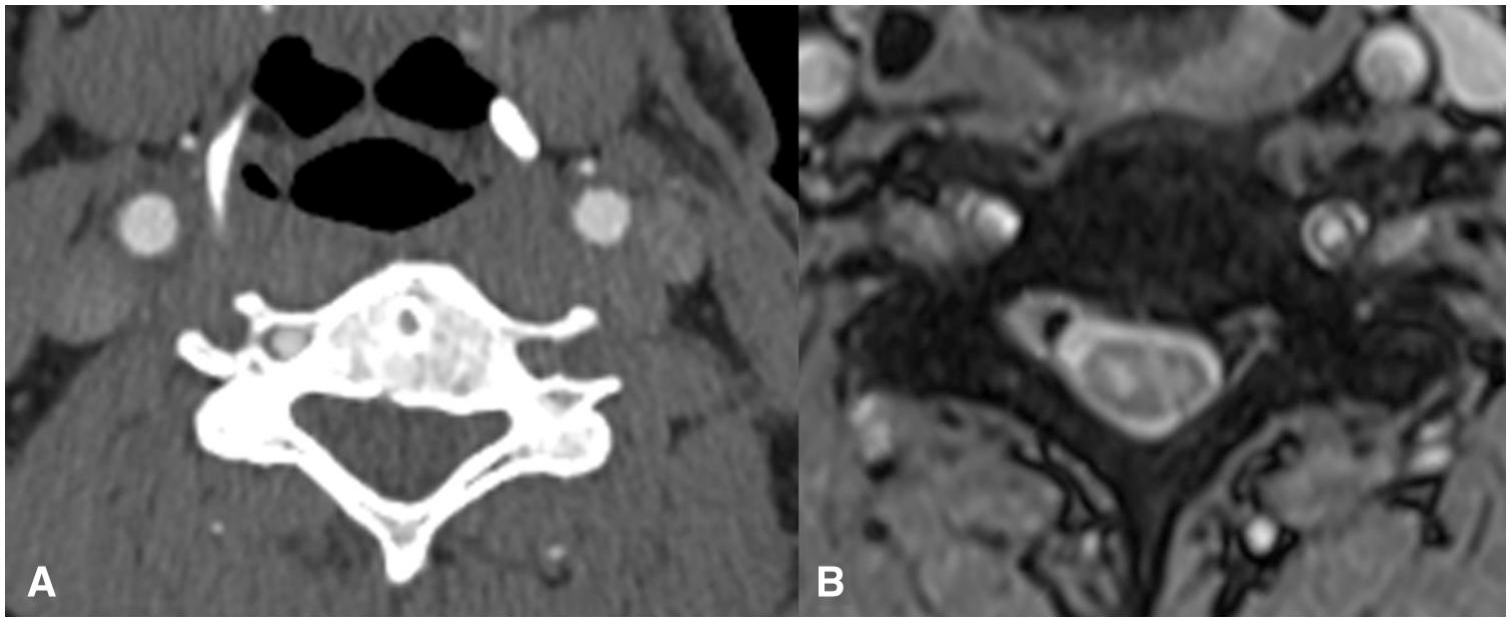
(A) CTA of the neck demonstrating loss of flow in the left vertebral artery. (B) Axial T2 MRI at C5-6 demonstrating right anterior SCI. The concurrent faint brightness, which is less pronounced in the left hemi-cord, is not represented on alternative views and was concluded to be artifactual at the multidisciplinary neuroradiology meeting.
